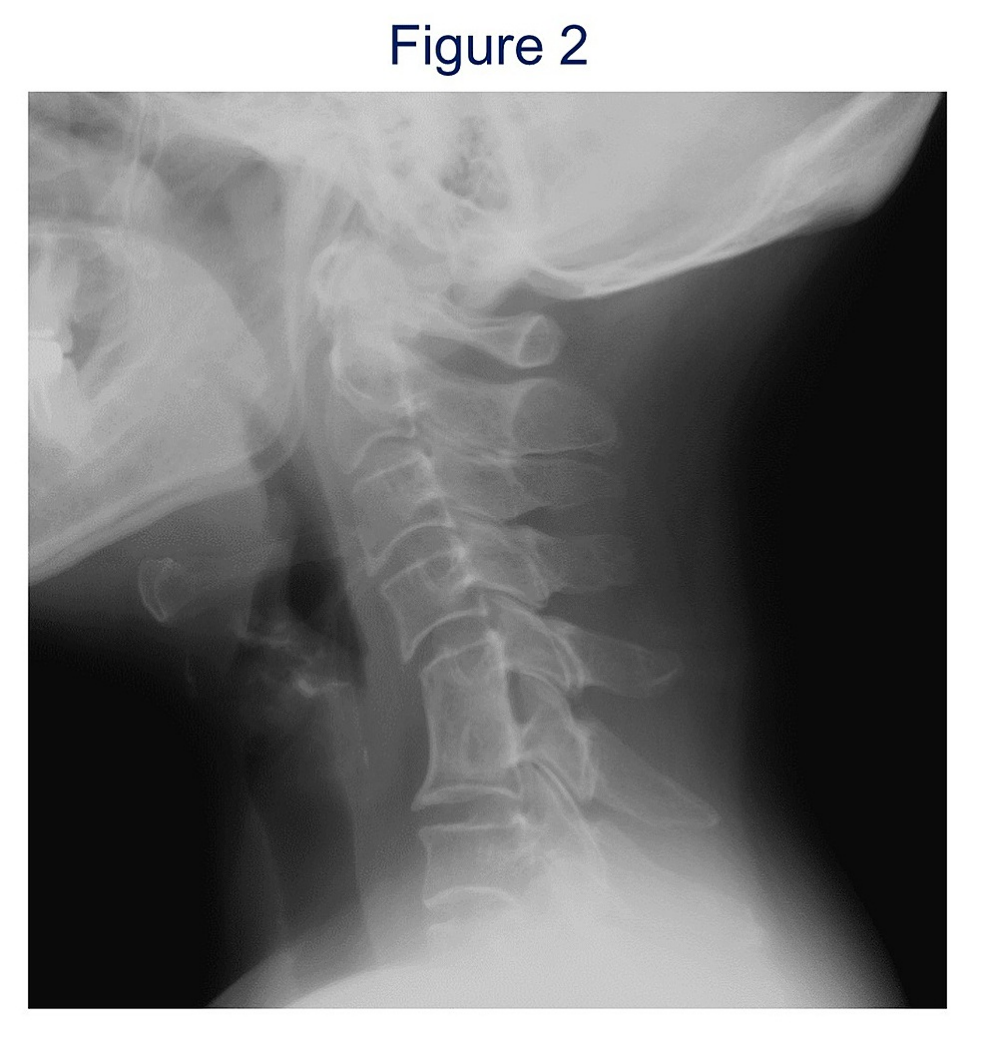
Postoperative lateral radiograph of case 1 (13 years after the surgery). Anterior cervical discectomy and fusion of C5-6 with an autogenous ilium bone graft without instrumentation was performed.
